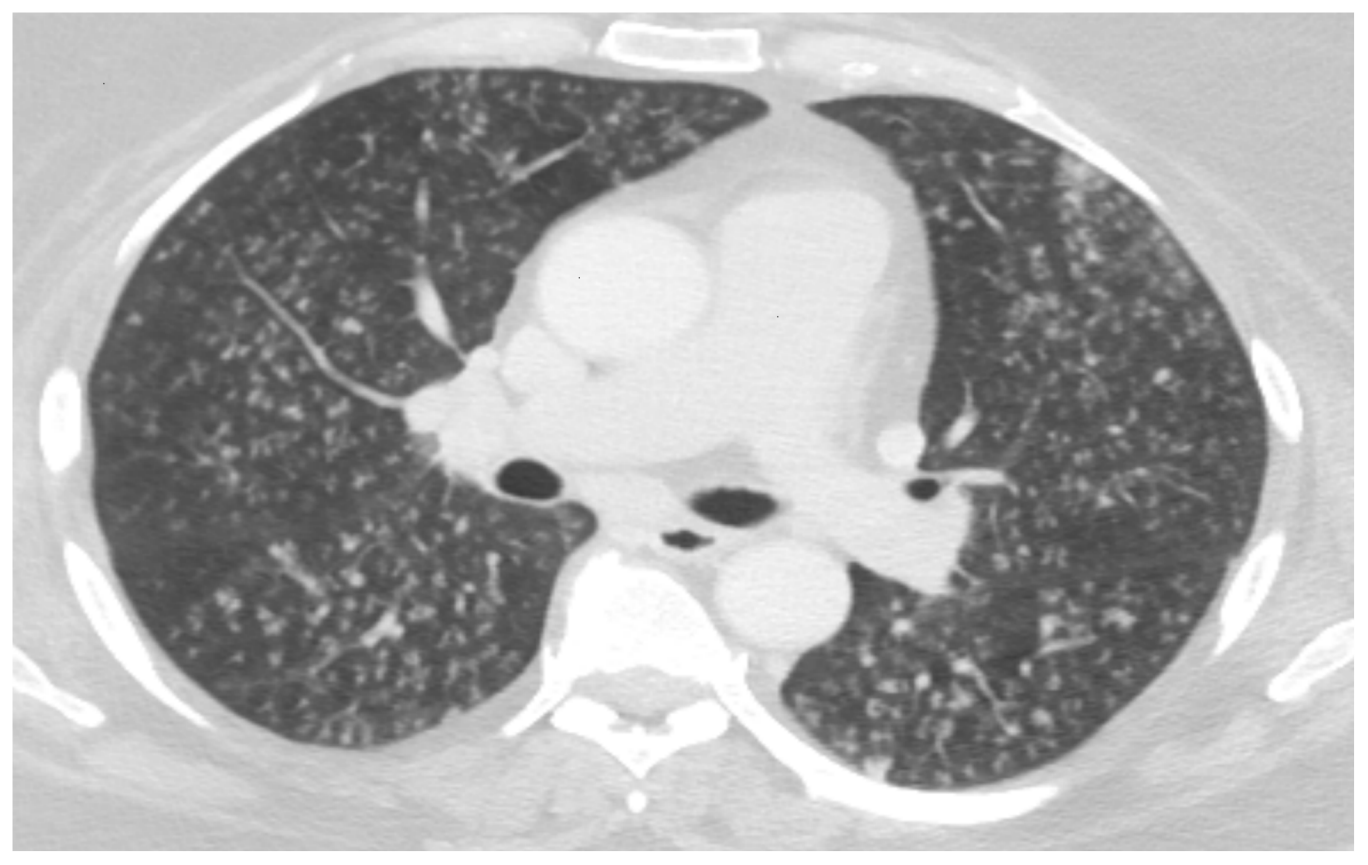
High-resolution chest CT image of a 57-year-old woman with acute bronchiolitis caused by respiratory syncytial virus. Numerous centrilobular nodules are present in both lungs.
